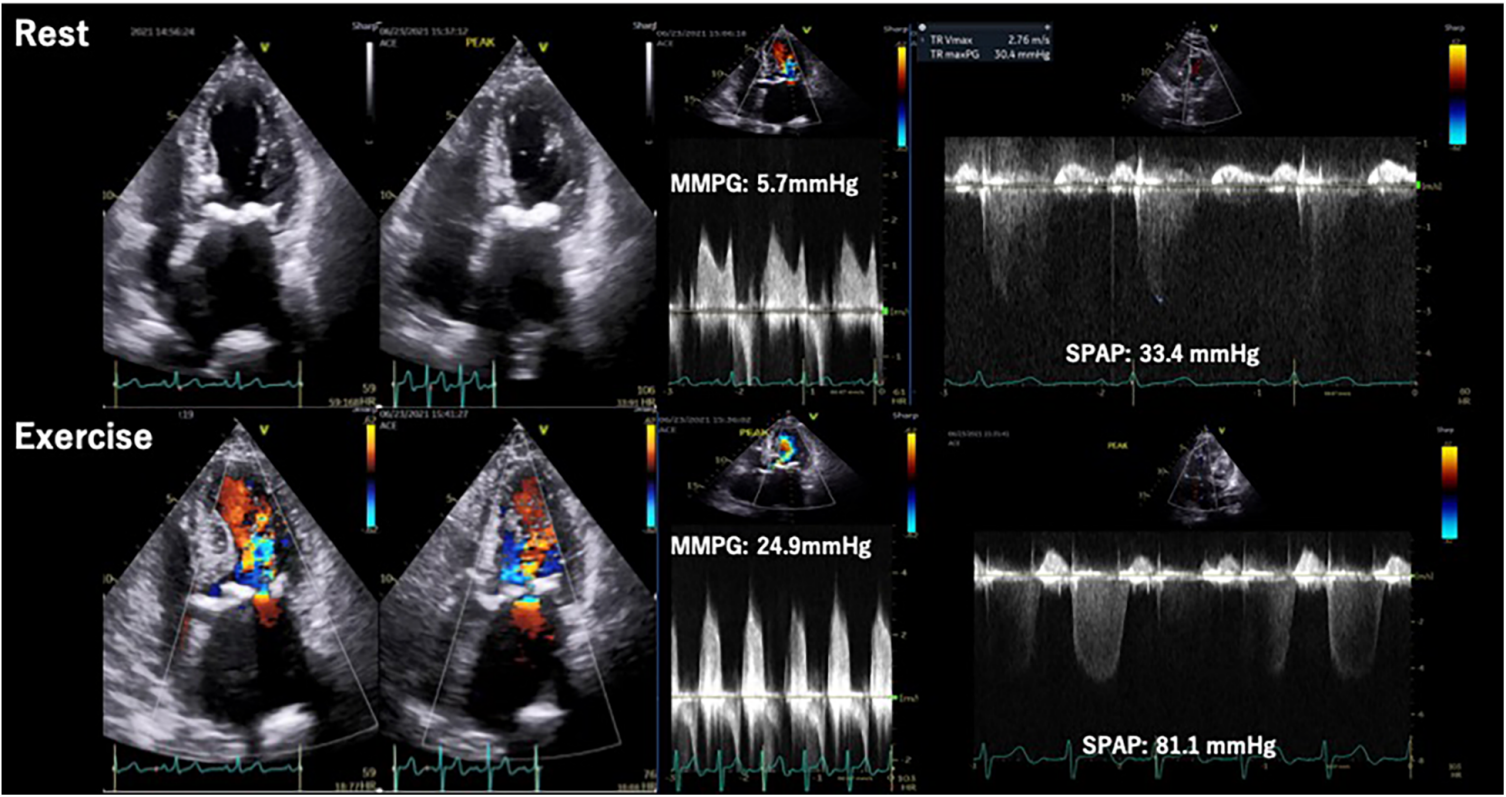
Stress echocardiography for prosthetic valve. Eighty-five-year-old man, a case of PPM after TAVI. Exercise stress induced increase of transprosthetic MPG, SPAP and worsening of diastolic dysfunction. MPG, mean pressure gradient; PPM, patient prosthesis mismatch; SPAP, systolic pulmonary arterial pressure; TAVI, transcatheter aortic valve implantation.
